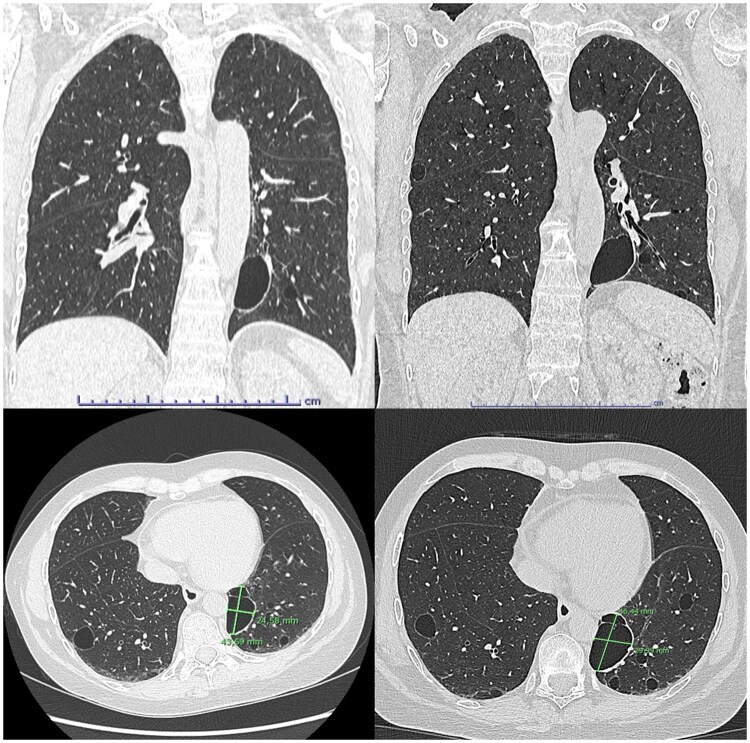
Images from a 50-year-old female patient with SjD-LIP. Top row: baseline and follow-up (after 32 months) coronal CT sections showing an increased number of cysts. Bottom row: measurements of the largest cyst, with diameters of 44 × 25 mm at baseline and 46 × 30 mm at follow-up
